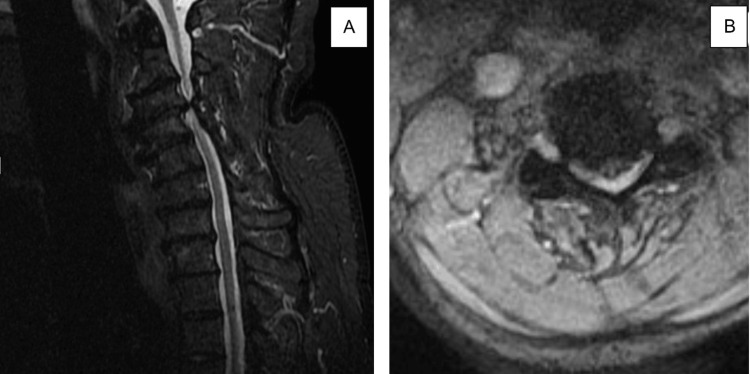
MRI of the cervical spine demonstrating severe cervical stenosis at C3-C4. (A) Sagittal. (B) Coronal.
